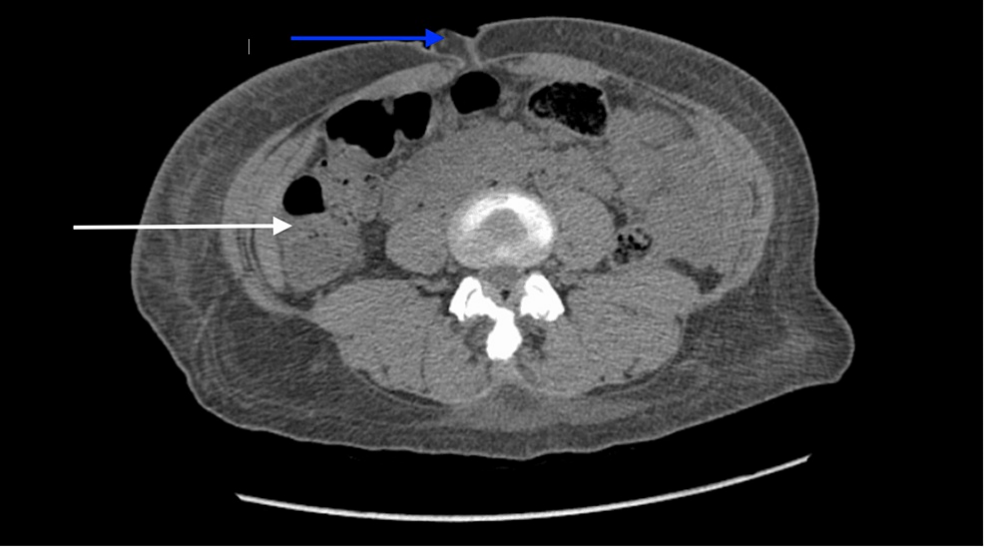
CT scan of the abdomen and pelvis without contrast. White arrow: Stool retention visualized in intestine; Blue arrow: Umbilical hernia.
